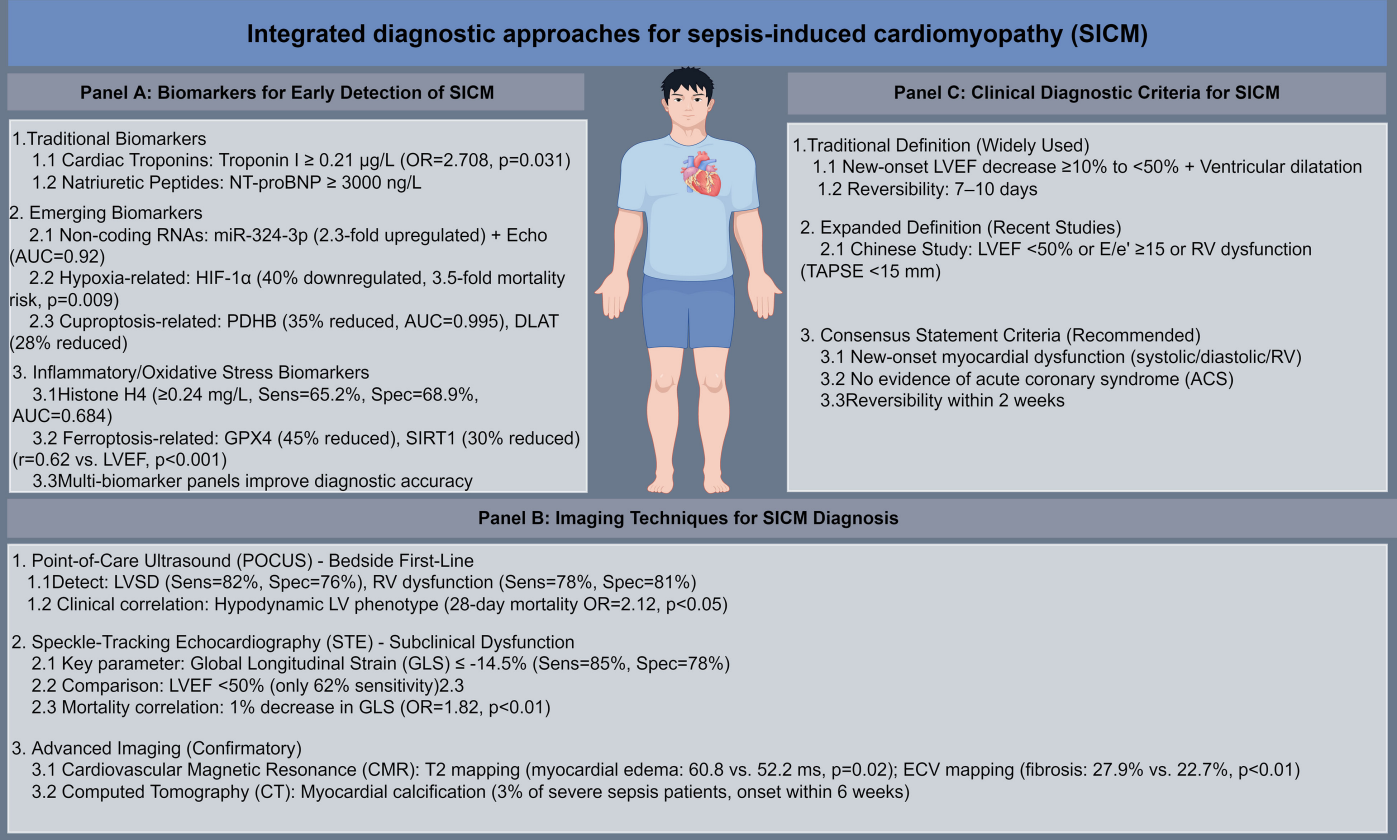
Integrated diagnostic approaches for sepsis-induced cardiomyopathy (SICM). (A) Key biomarkers for early detection of SICM, categorized into traditional markers (cardiac troponins, natriuretic peptides), emerging markers (non-coding RNAs, HIF-1α, cuproptosis-related proteins), and inflammatory/oxidative stress markers (histone H4, ferroptosis-related proteins). Critical cut-off values, fold changes, and diagnostic performance (AUC, OR, *p*-values) are indicated. (B) Hierarchical imaging techniques for SICM diagnosis, including bedside point-of-care ultrasound (POCUS) as first-line assessment, speckle-tracking echocardiography (STE) for subclinical myocardial dysfunction, and advanced cardiovascular magnetic resonance (CMR) and computed tomography (CT) for tissue characterization. Sensitivity, specificity, and clinical correlations are provided. (C) Evolution of clinical diagnostic criteria for SICM, from the traditional definition (LVEF decrease + ventricular dilatation) to expanded definitions (including diastolic and right ventricular dysfunction) and consensus criteria (three core requirements).
